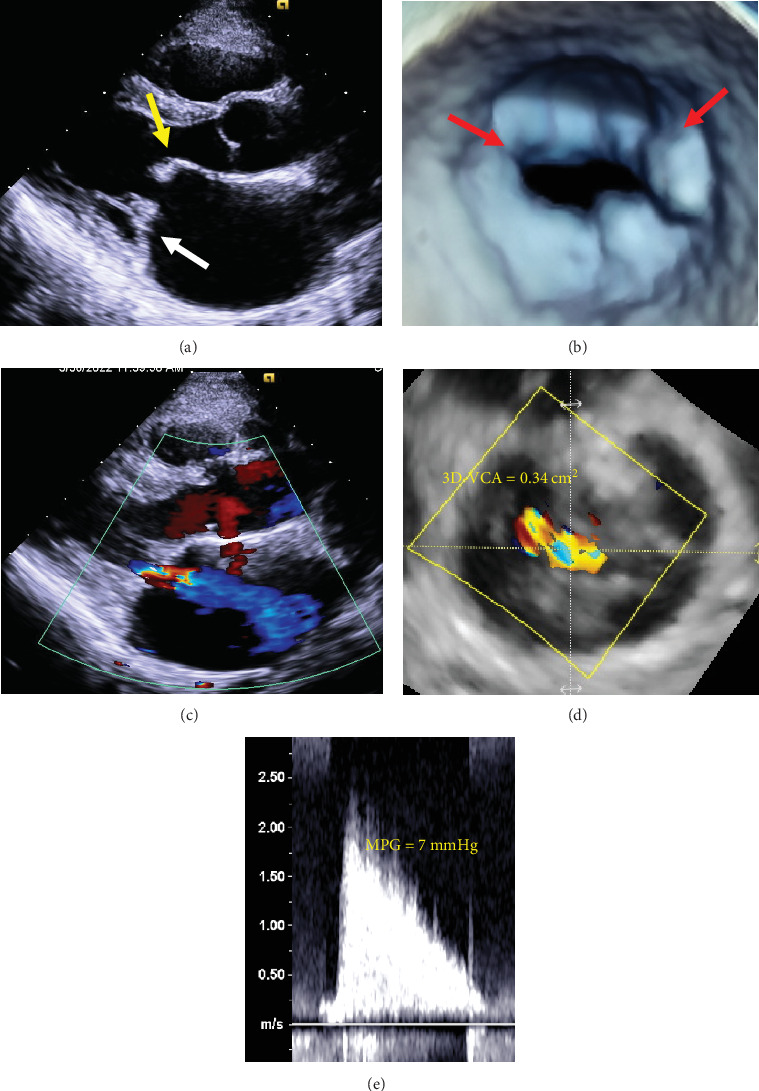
Two-dimensional (2D) TTE, parasternal long-axis view (a), and three-dimensional (3D) TEE, en face view from the left atrium (b), demonstrate the “hockey stick” appearance of the anterior leaflet (*yellow arrow*), poor mobility of the posterior leaflet (*white arrow*), and fusion of the commissures (*red arrows*). Continuous-wave Doppler across a stenotic mitral valve by TTE (c). Mean pressure gradient of 7 mmHg indicates moderate MS. 2D TTE color Doppler (d) and 3D TEE multiplanar reconstruction imaging (e) at mid-systole demonstrate central moderate MR. MPG, mean pressure gradient; 3D-VCA, three-dimensional vena contracta area.
